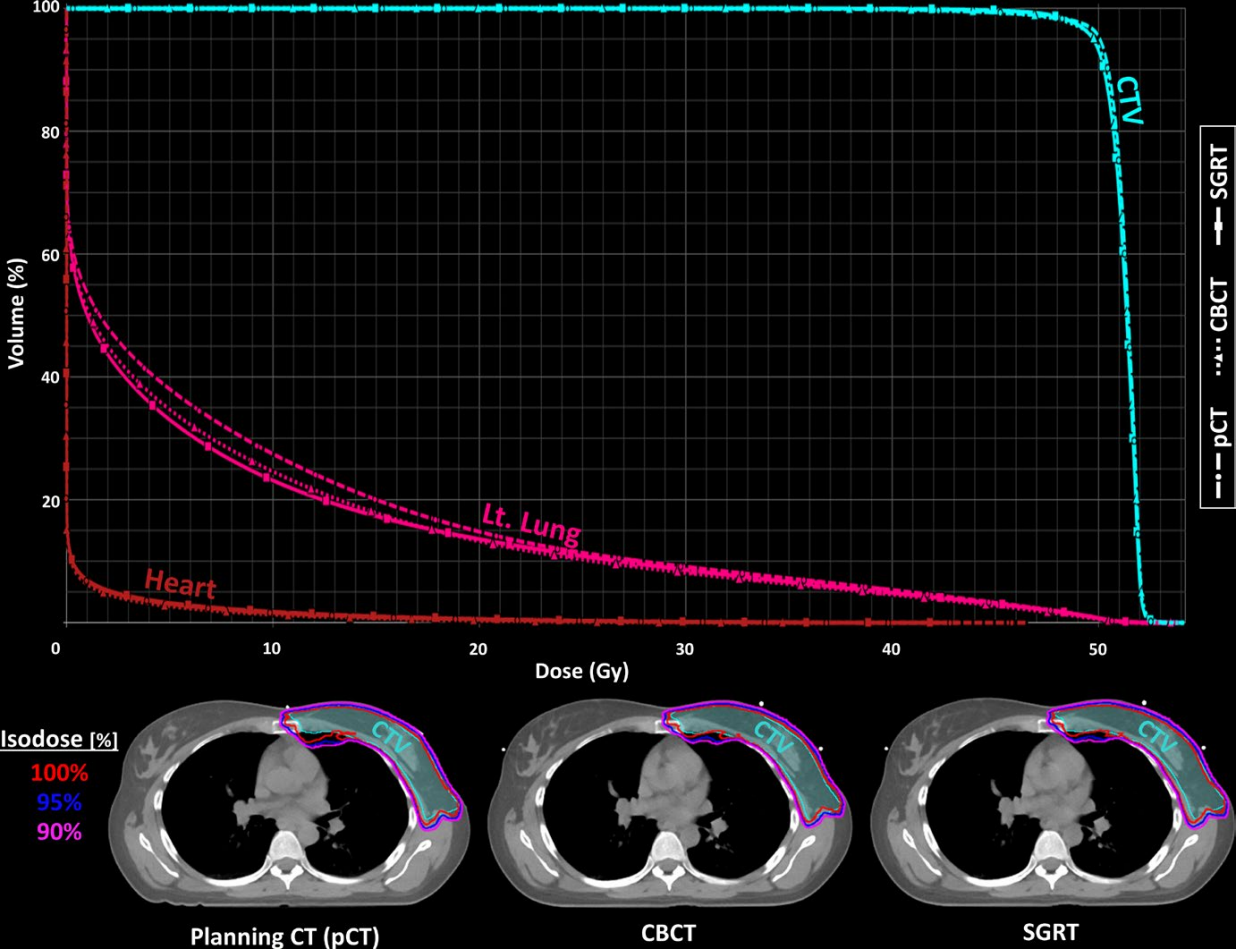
Top: Sample dose–volume histogram Bottom: Dose distributions from the planning CT (left) and the QA‐CT registered using cone‐beam computed tomography (CBCT)‐like (middle) and surface‐guided radiation therapy (SGRT)‐like (right) techniques
